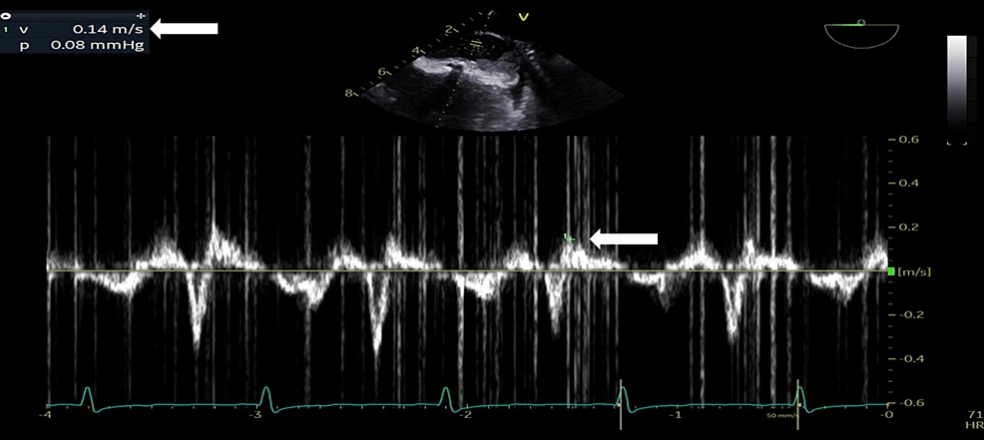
Transesophageal echocardiography: pulsed wave Doppler at the opening of the left atrial appendage. Note the low left atrial appendage velocity of 14 cm/s (arrow).
